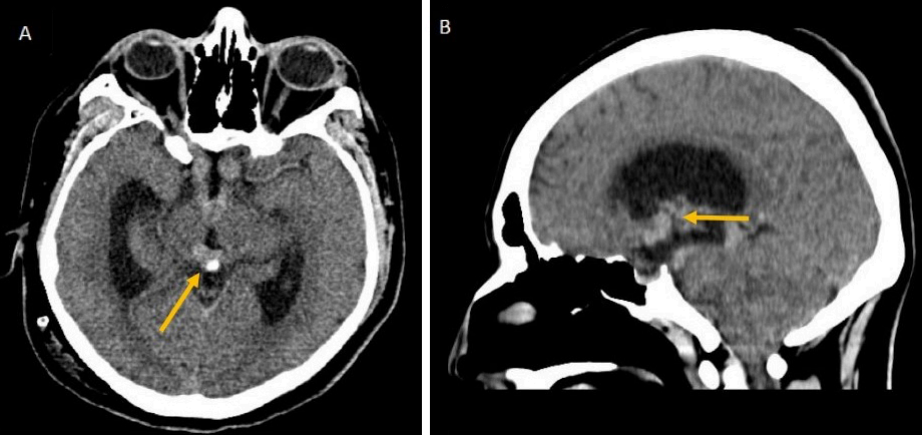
Brain computed tomography findings. A. Axial nonenhanced computed tomography (NECT) scan shows typical findings of pineal germinoma with a well-demarcated slightly hyperdense tumor engulfing the calcified pineal gland. B. Sagittal NECT scan shows a lobulated hyperdense mass with different locations, suprasellar, infundibular, and tumor spread, in the lateral ventricles and third ventricle with hydrocephalus.
